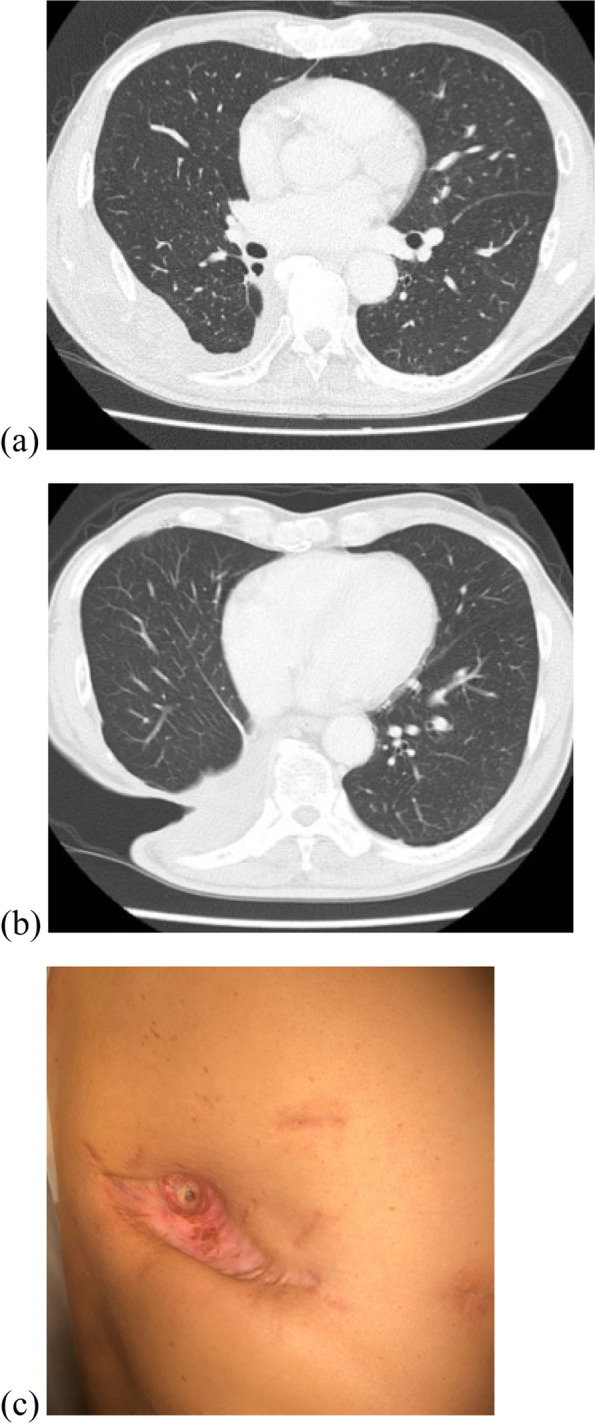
a, b CT scan of the thorax shows the expansion of the residual lung, and there are no signs of the fistula. c Appearance of the right lateral chest. The bottom of the open-window thoracostomy was observed
